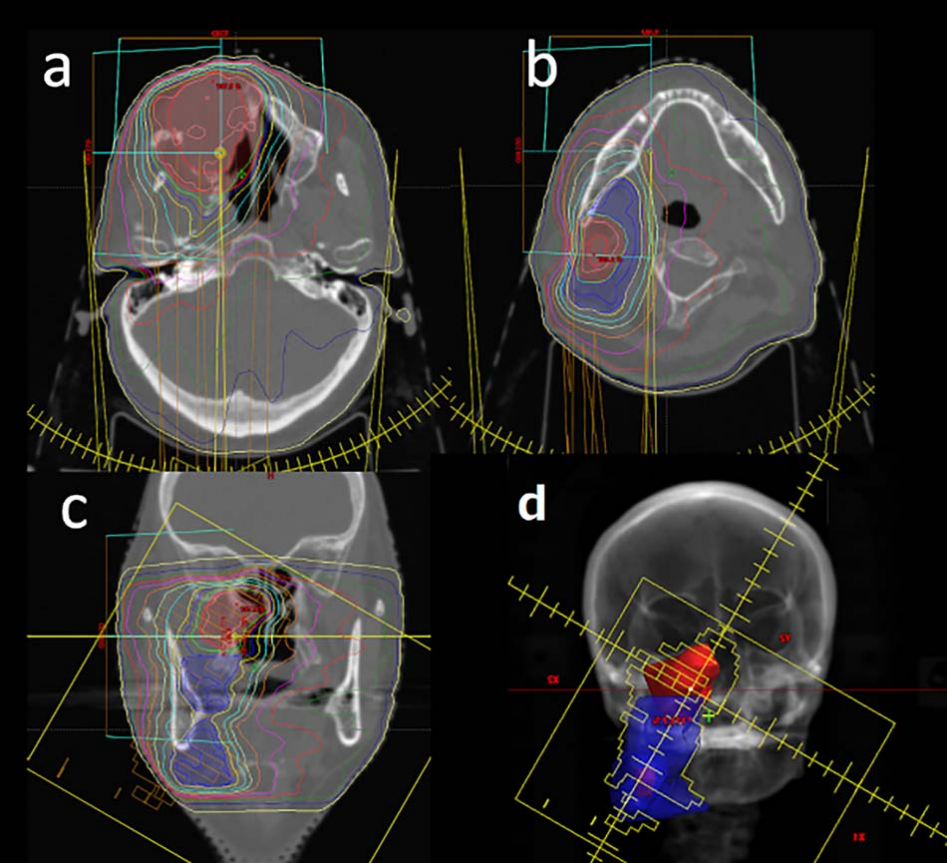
A representative case treated with intensity modulated radiotherapy is shown. (a) Axial CT image at the level of primary tumor. (b) Axial CT image at the level of lymph node metastasis. (c) Coronal CT image. (d) Beam’s eye view of the treatment plan. The primary tumor is shown in red mesh and the neck lymph node area is shown in blue mesh. Blue line, 95% isodose line of prescribed dose.
